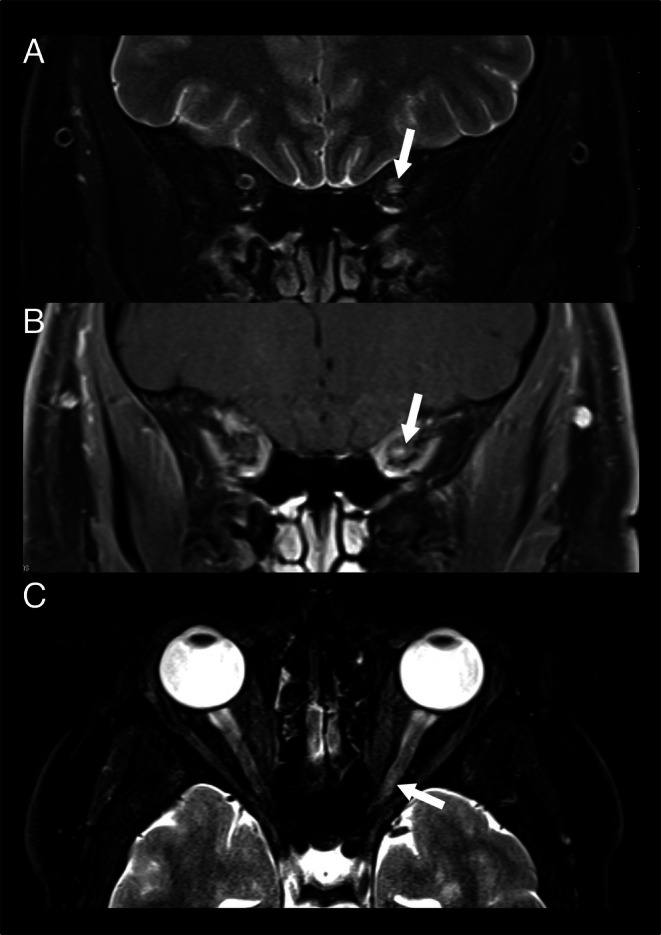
Optic nerve imaging included a (A) coronal T2 TSE with fat saturation, a (B) coronal T1 TSE with fat saturation as well as a (C) axial T2 TSE with fat saturation. In this 26‐year‐old male patient, a clinically acute optic neuritis was confirmed with MR imaging (arrows). In other cases, the signal alterations within the optic nerve were only subtle, leading to different interpretations by the readers.
